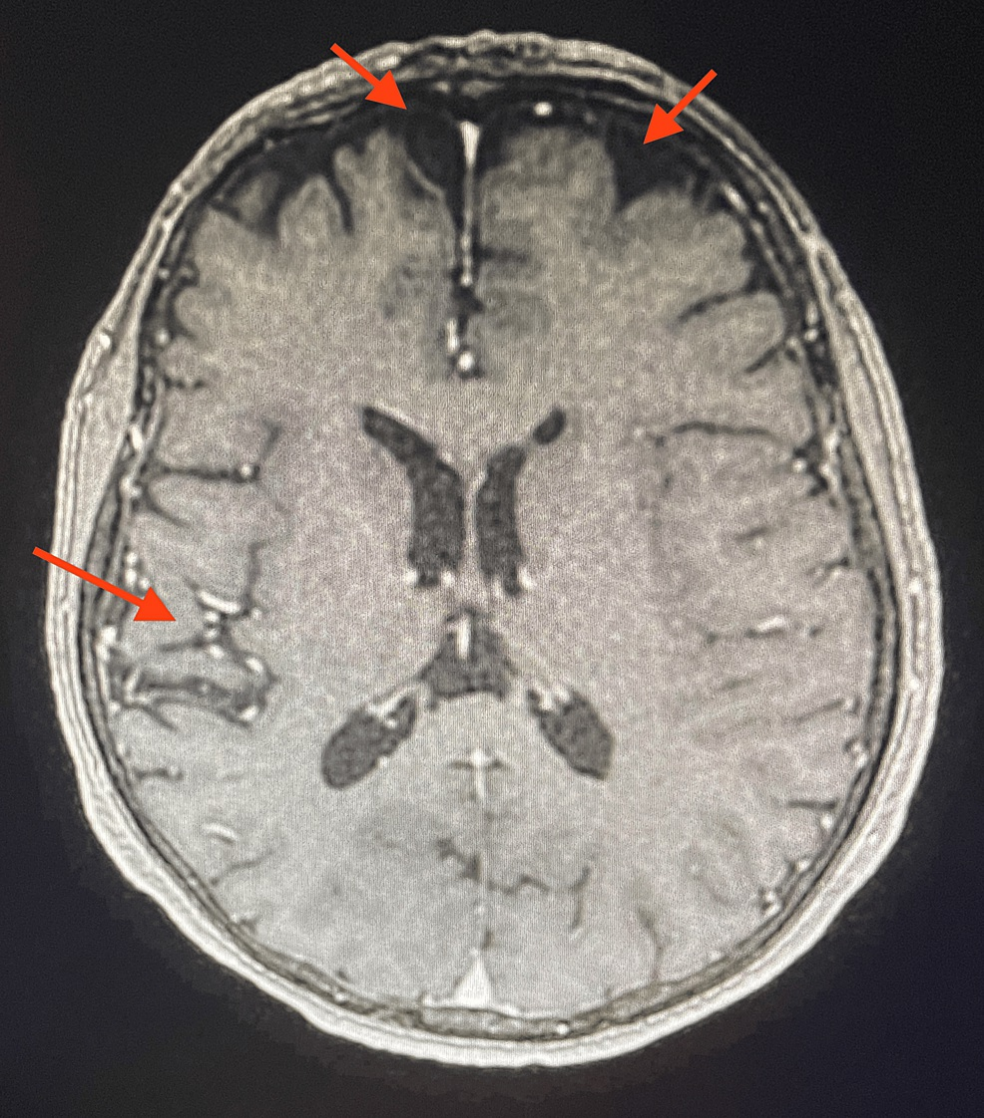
MRI with and without contrast ordered during admission. MRI showed evidence of mild frontotemporal atrophy and microangiopathy (red arrows). No acute infarcts, masses, or mass effects were observed.
